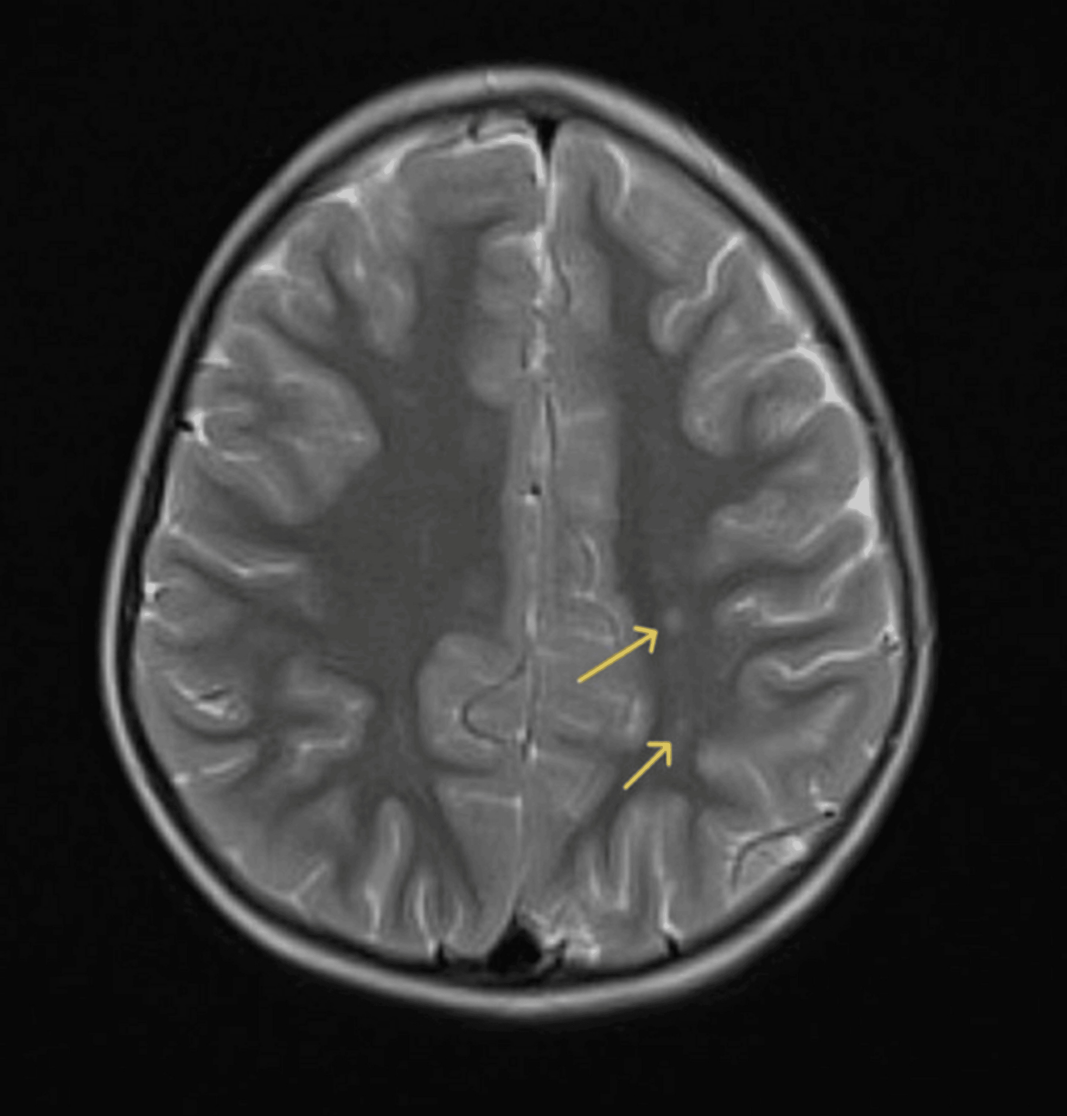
Follow-up MRI scan Follow-up axial T2-weighted MRI showing stable parasagittal dilated perivascular spaces in bilateral parietal white matter (arrows), without interval progression.
